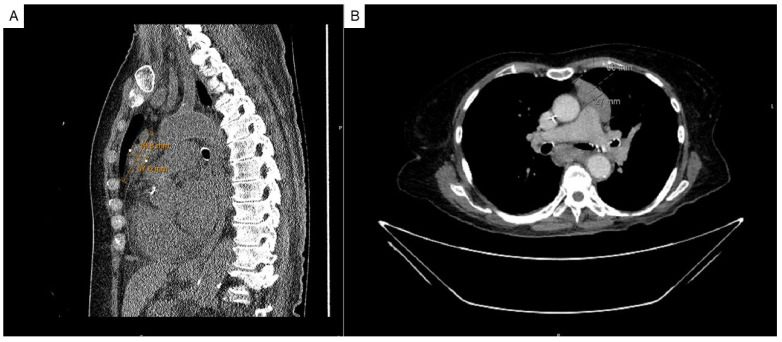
(A) Sagittal and (B) axial views of the CT scan of the chest (without contrast), demonstrating a large, lobulated anterior mediastinal mass measuring 6.6 × 3.5 × 7.5 cm abutting the left anterolateral aspects of the ascending aorta and main pulmonary artery.
